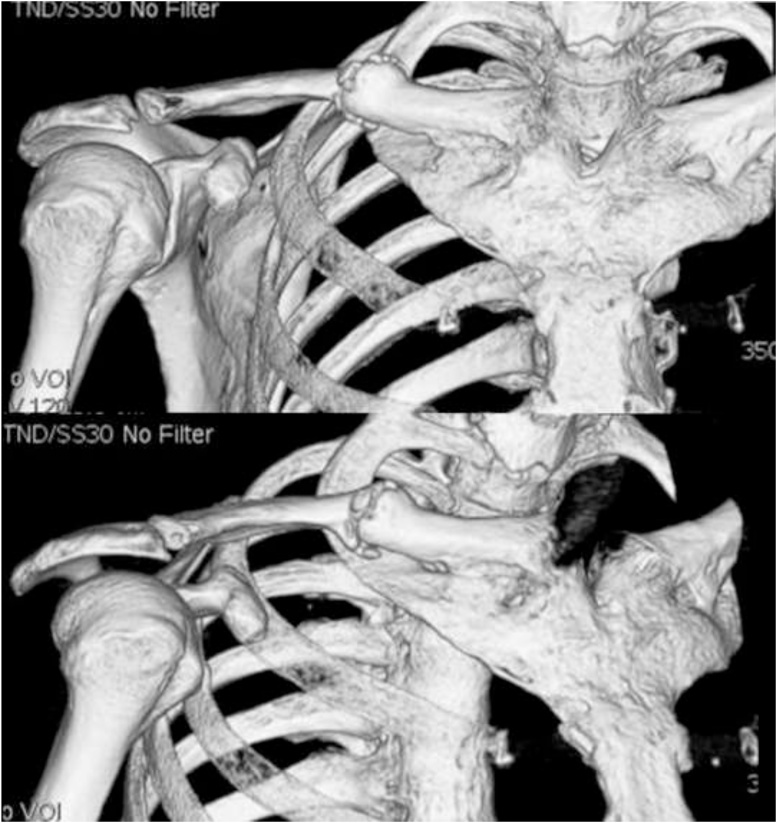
Anteroposterior three-dimensional computed tomography of the clavicle included the sternoclavicular joints.
